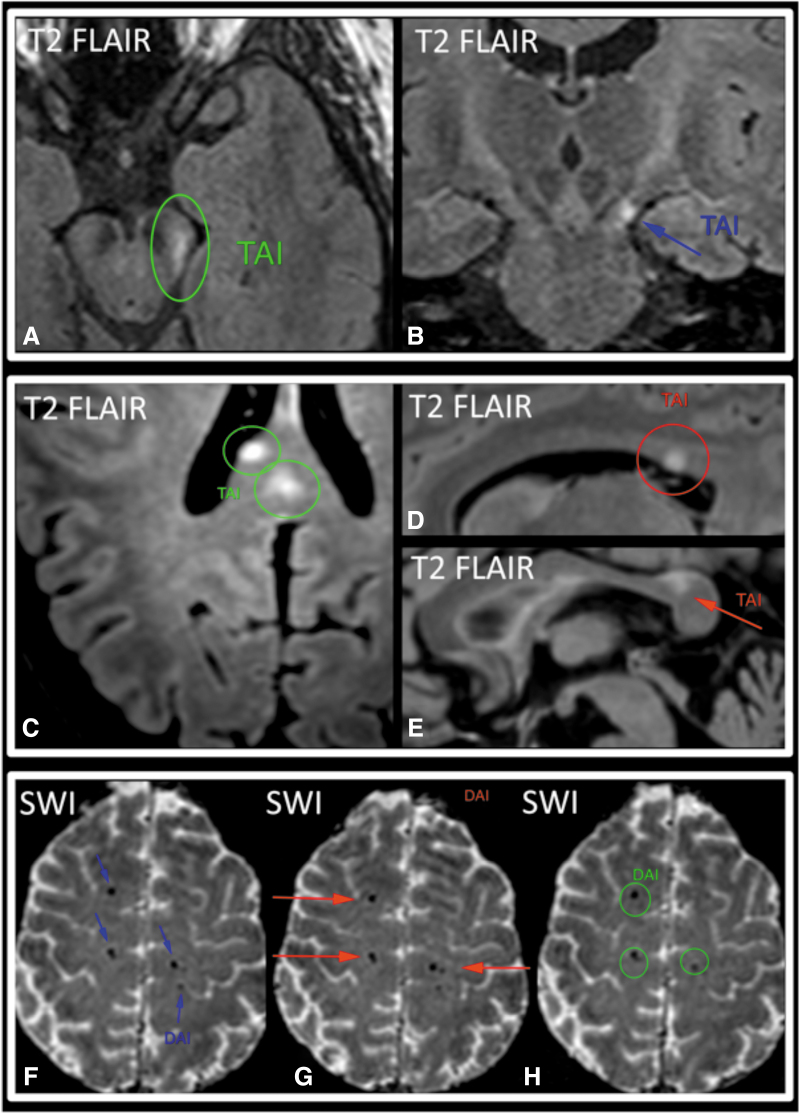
Traumatic axonal injury (TAI) and diffuse axonal injury (DAI). Two examples of partial (A,B, C–E) and one example of unanimous agreement (F–H) across three neuroradiologists. “Partial agreement” refers to MRI exams for which the three readers' initial interpretations regarding presence of TAI/DAI on the exam were not unanimous. Annotations from independent readers are shown in different colors (red, green, and blue) superimposed on the same image for demonstration purposes. (A,B) Single focus of T2-weighted FLAIR hyperintensity in the left cerebral peduncle (without susceptibility artifact), consistent with TAI. (C–E) Two foci of T2-weighted FLAIR hyperintensity in the body and splenium of the corpus callosum (without susceptibility artifact), also consistent with TAI. (F–H) Multiple (more than four) foci of susceptibility artifact in more than one lobe of the brain on T2*-weighted series (SWI), consistent with DAI. Additional foci marked by readers on other slices are not shown. FLAIR, fluid-attenuated inversion recovery; MRI, magnetic resonance imaging; SWI, susceptibility-weighted imaging.
